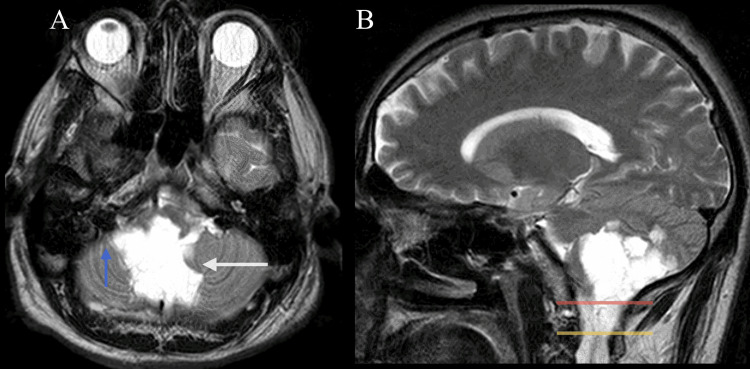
MRI of the posterior fossa lesion. A: Axial T2-weighted MRI demonstrates a large, well-circumscribed hyperintense lesion within the posterior fossa, involving the cerebellar vermis (white arrow) and right cerebellar hemisphere, with extension into the right cerebellopontine angle (blue arrow). The lesion exerts mass effect on adjacent cerebellar structures without evidence of obstructive hydrocephalus. B: Sagittal T2-weighted MRI shows inferior extension of the lesion through the foramen magnum (red line) into the upper cervical spinal canal (yellow line), with associated compression of posterior fossa structures.
